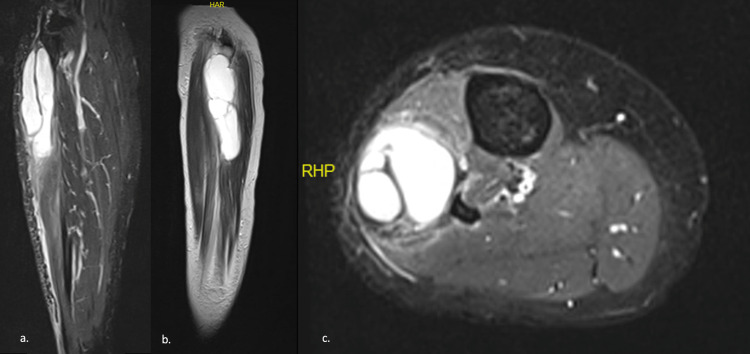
(a) Coronal, (b) sagittal, and (c) axial T2-weighted MRI images of the ganglion cyst
